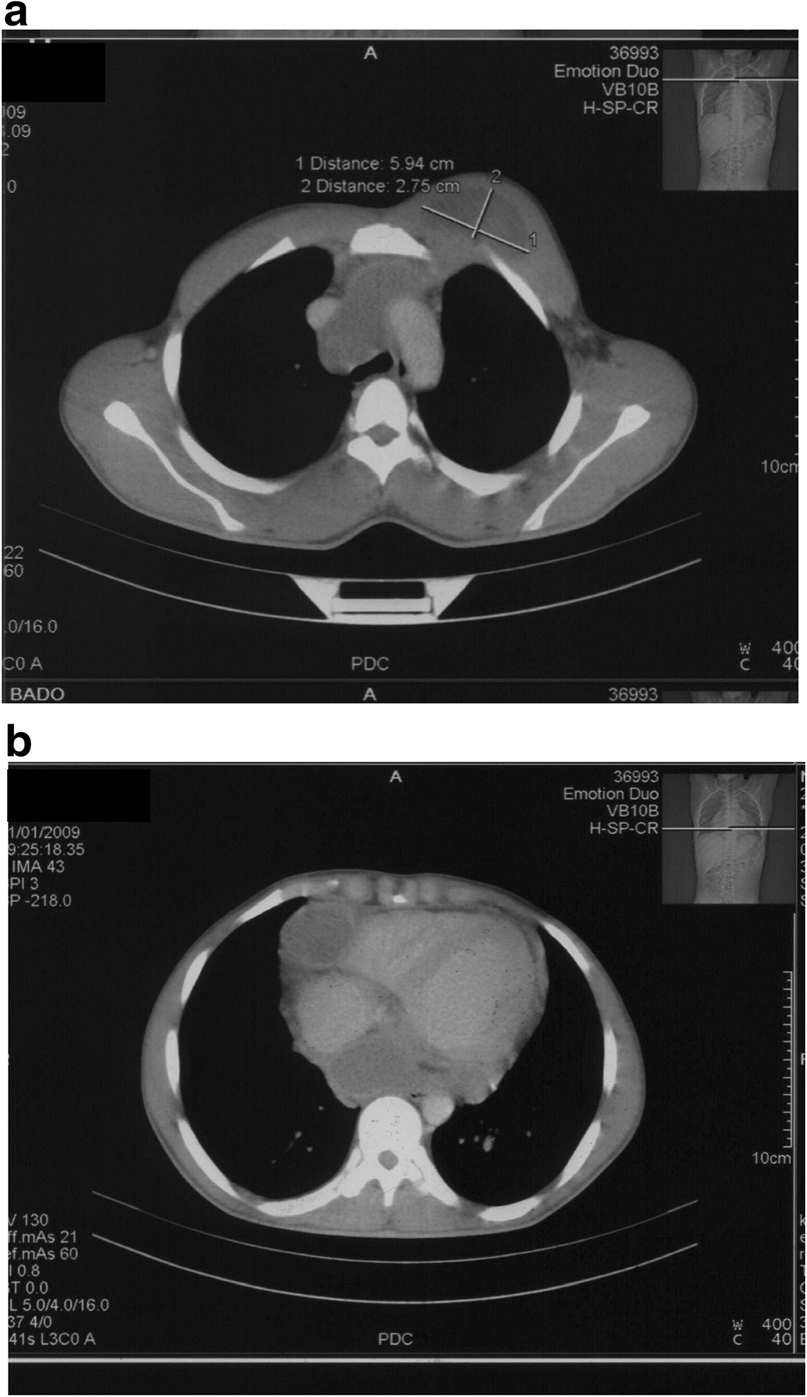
Chest CT scan at presentation. a. Mass visualized in left anterior chest wall measuring 5.9 cm×2.7 cm. b. Pericardial mass visualized around the right atrioventricular junction.
